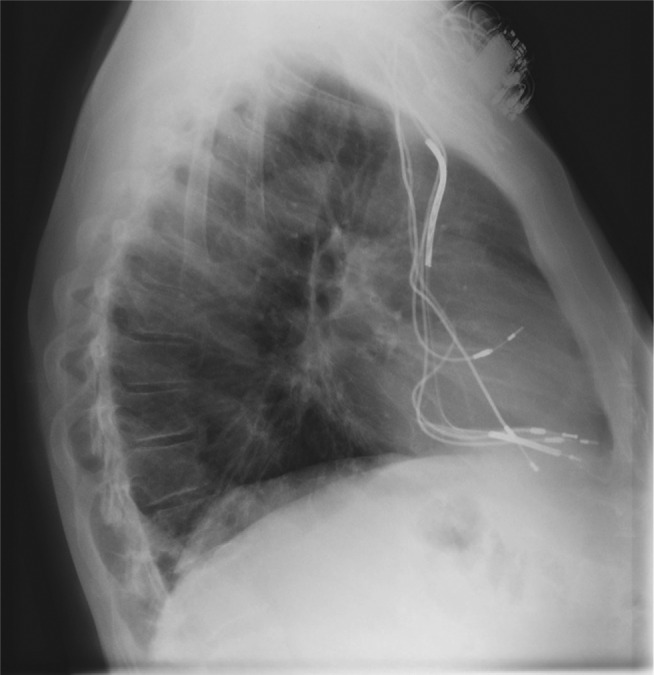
Initial lateral chest X-ray showing four leads crossing the tricuspid valve and causing severe TR.
